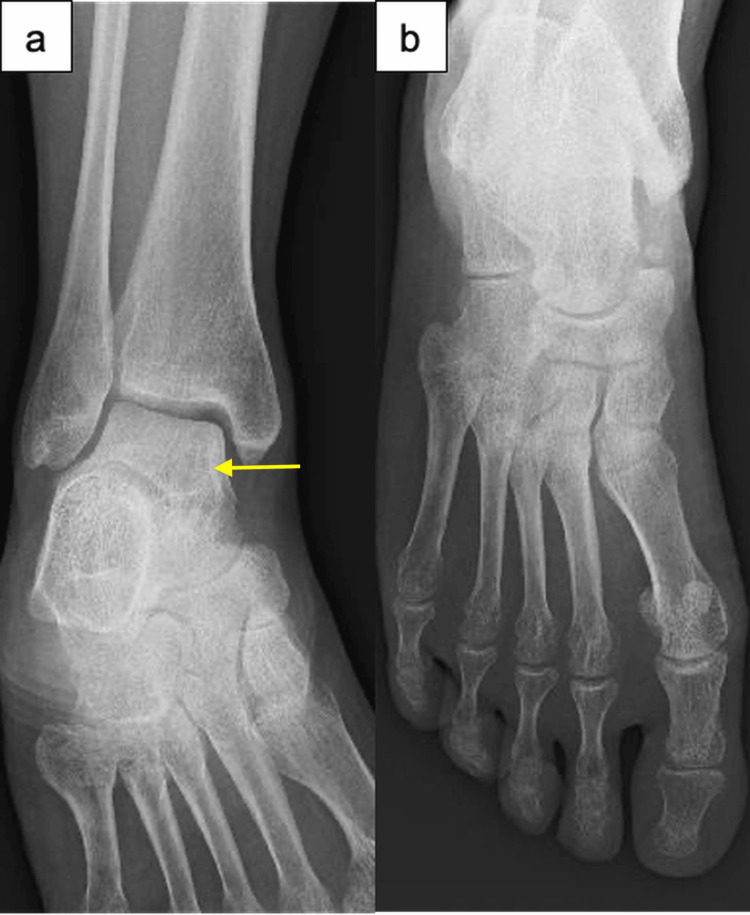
Serial anteroposterior radiographs of the right ankle and foot at eight months postpartum. At eight months postpartum: (a) complete normalization of bone density in the ankle; (b) complete normalization of bone density in the foot. The arrow indicates a representative area of normalized bone density in the talus.
